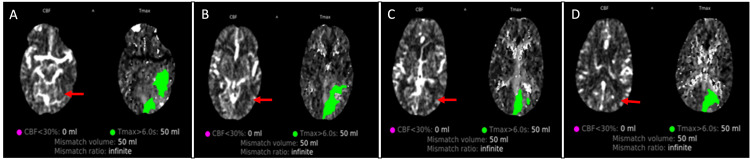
Case 2: Mismatch of CBF (ischemic core) and brain at risk (green) Advanced CT imaging shows a mismatch between normal CBF (red arrow) and perfusion (prolonged time to maximum flow (Tmax)) (green fill). The green area is the ischemic penumbra, or brain at risk. This mismatch triggered the administration of intravenous thrombolytic therapy to facilitate reperfusion. A-D are transverse sections of the brain on advanced CT imaging. CBF, cerebral blood flow
